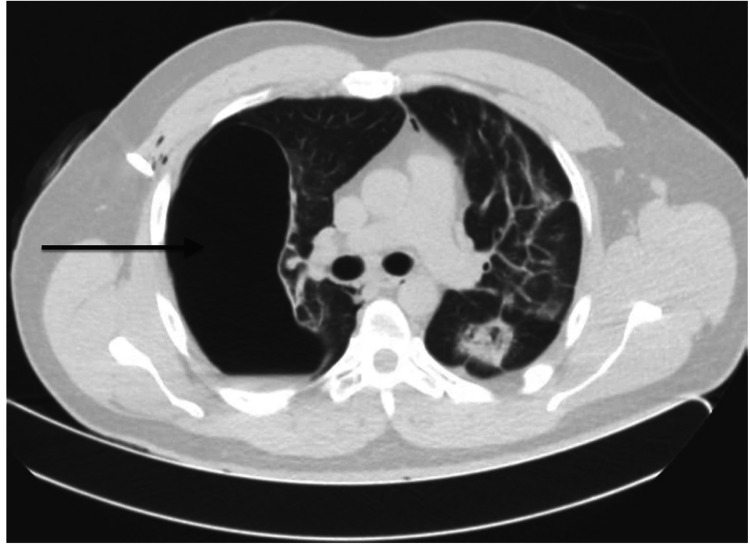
CT showing large right bullae (arrow)
